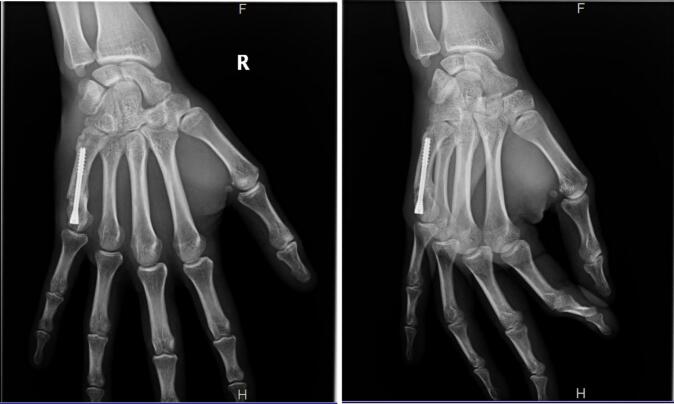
X-rays 6 weeks post-operative.
